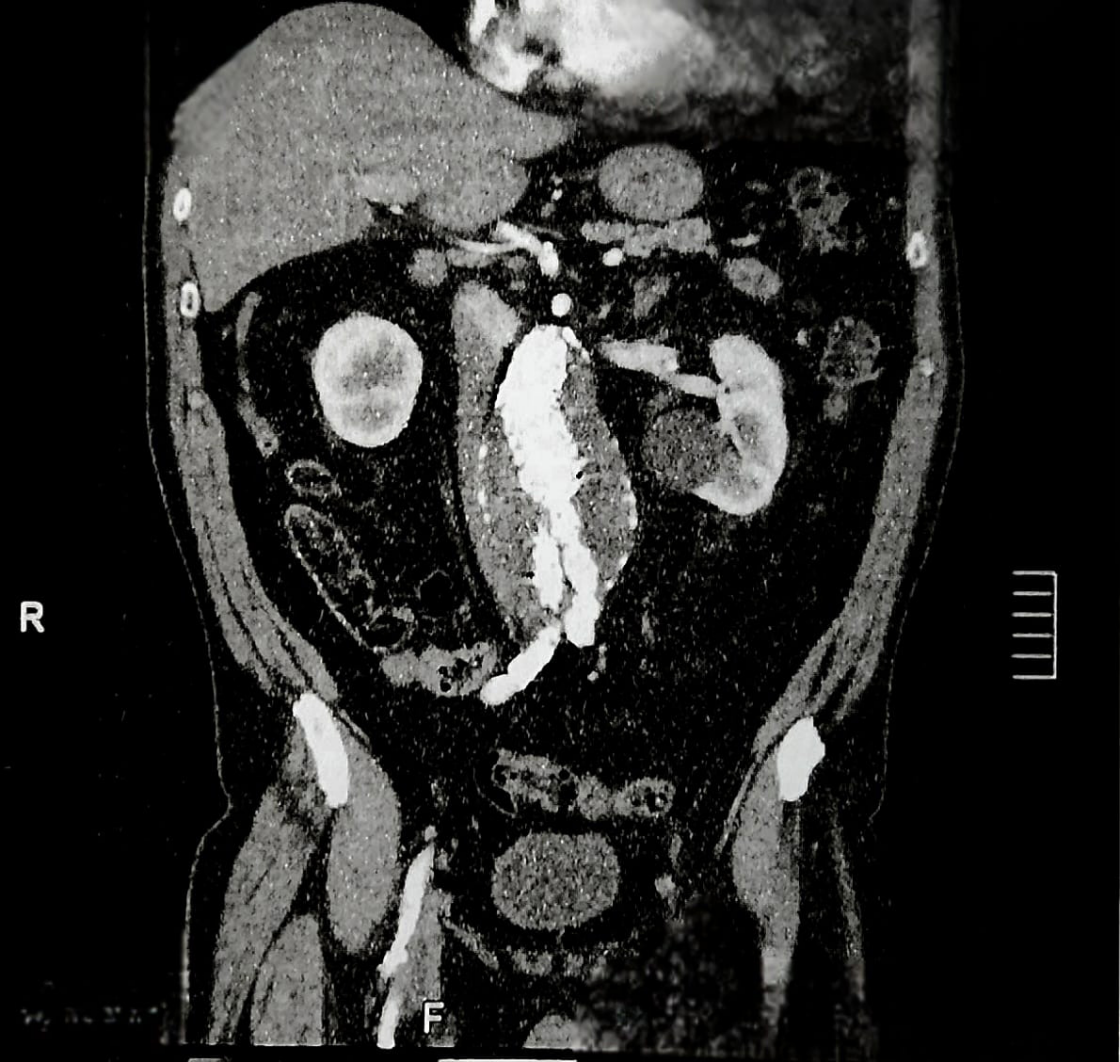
Postoperative computed tomography with contrast showing repair of the endoleak and maintenance of blood flow in the abdominal aorta, in 2016.
